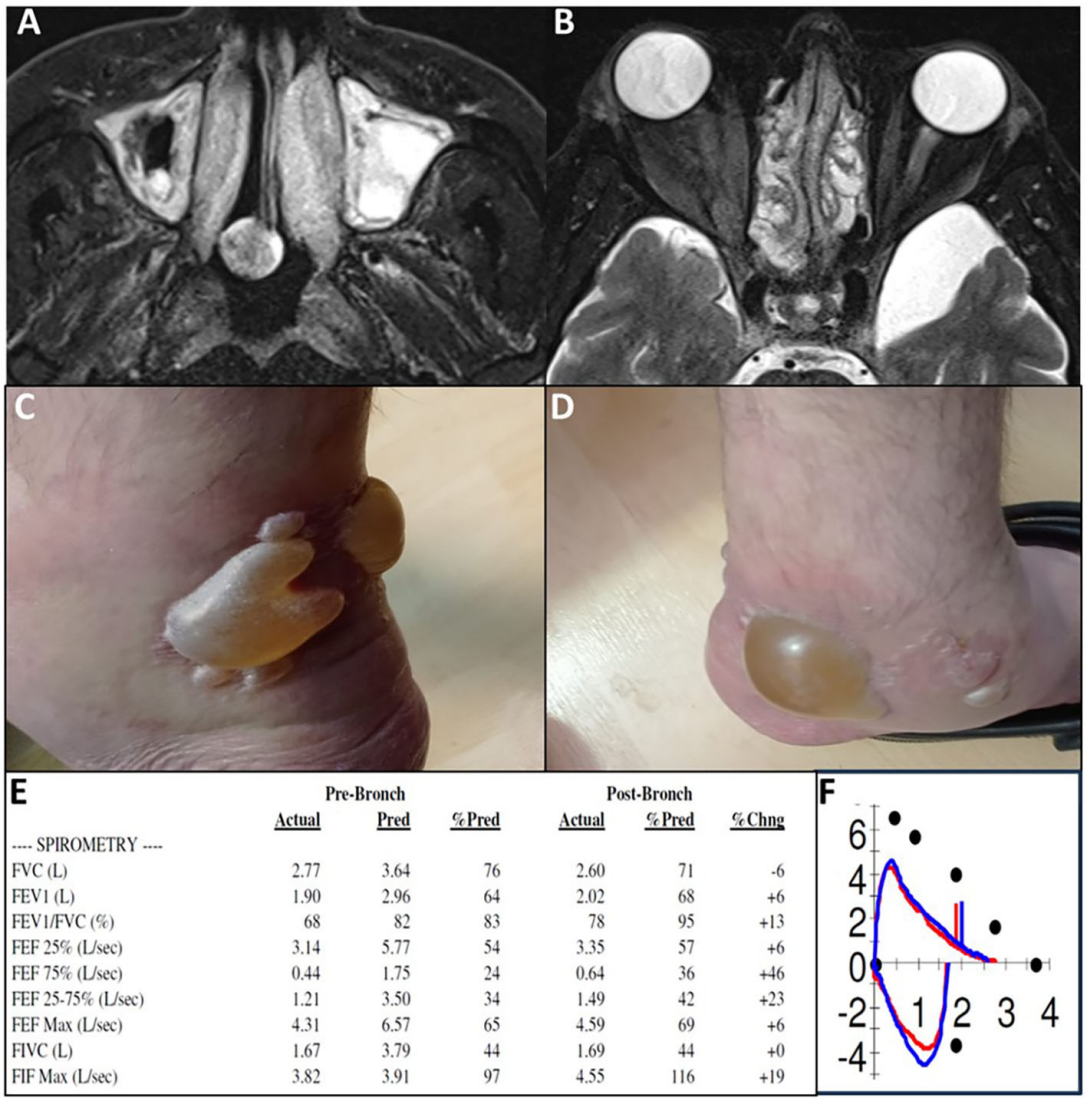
Recurrence of diseases following study completion and benralizumab discontinuation. (A, B) P15, eosinophilic sinusitis with nasal polyposis. MRI 2 months post-study shows recurrence of severe sinus disease (T2 axial images). (C, D) P17, severe EGPA with eosinophilic carditis. Bilateral ankle bullae observed 6 months post-study during EGPA exacerbation with worsening asthma and elevated inflammatory markers; fluid analysis shows 600 eosinophils. (E, F) P10, steroid-dependent chronic eosinophilic pneumonia. Pulmonary function tests 5 months post-study demonstrate combined obstructive and restrictive lung disease (FEV1/FVC 68%, FEV1 64% predicted, FVC 76% predicted). Flow-volume loop (F) shows a concave expiratory limb indicating obstruction and reduced loop size indicating restriction, consistent with PFT results.
